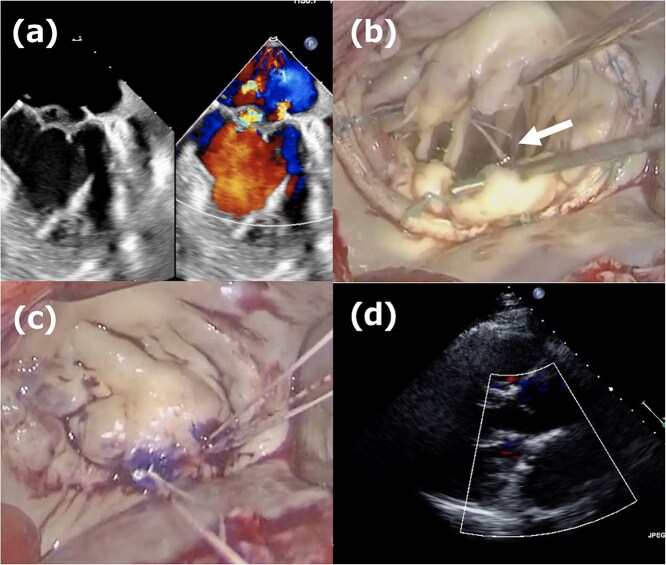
Second operation images. (a) Moderate mitral regurgitation caused by partial prolapse of the A2 segment, observed 3 weeks after the initial operation. (b) Intraoperative image showing the rupture of the ePTFE loop at the fixation site onto the leaflet during reoperation. (c) Three 20-mm long CV4 loops were attached to the posterior papillary muscle, and those loops were fixed to the A2 segment with CV4. (d) One week postoperative echocardiogram showing no residual mitral regurgitation after reoperation.
